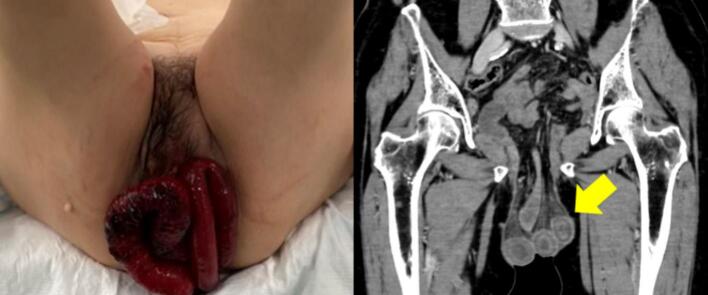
Physical examination: Evisceration of dark red small intestine was observed from the urethral extirpation site. The abdominal contrast-enhanced computed tomography scan findings: the arrow indicates thickened ileum wall and oedematous mesentery.
